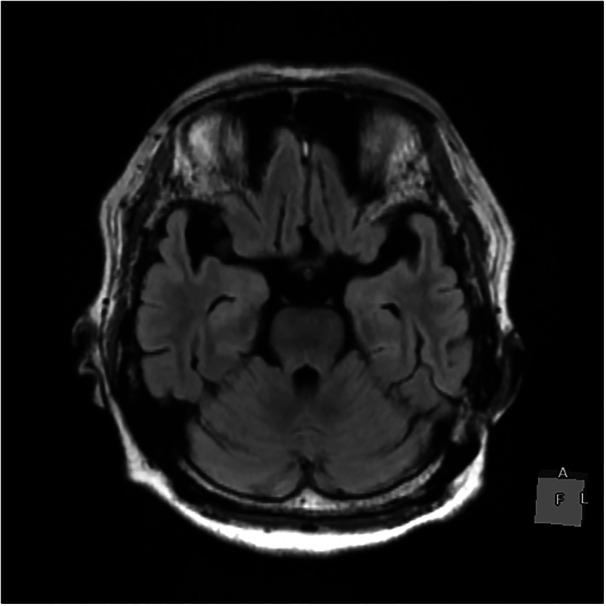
Brain magnetic resonance imaging revealed no lesions that could account for the catatonic state.
